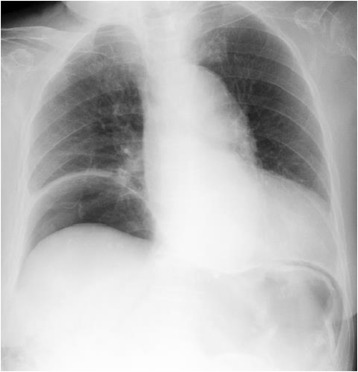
Chest radiograph in the sitting posture. Chest radiograph shows free gas in the right and left subdiaphragmatic regions
